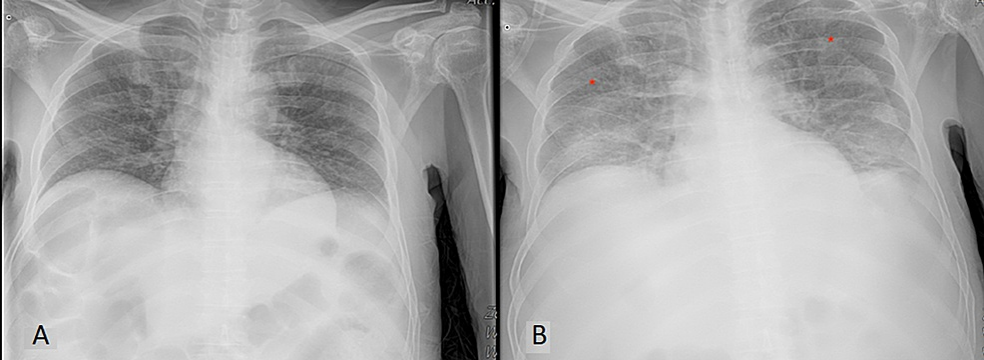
(A) Chest x-ray from the previous admission displays no abnormalities; (B) chest x-ray displays subpulmonic effusions near diaphragm area, and the asterisks display increased congestion and infiltrate
